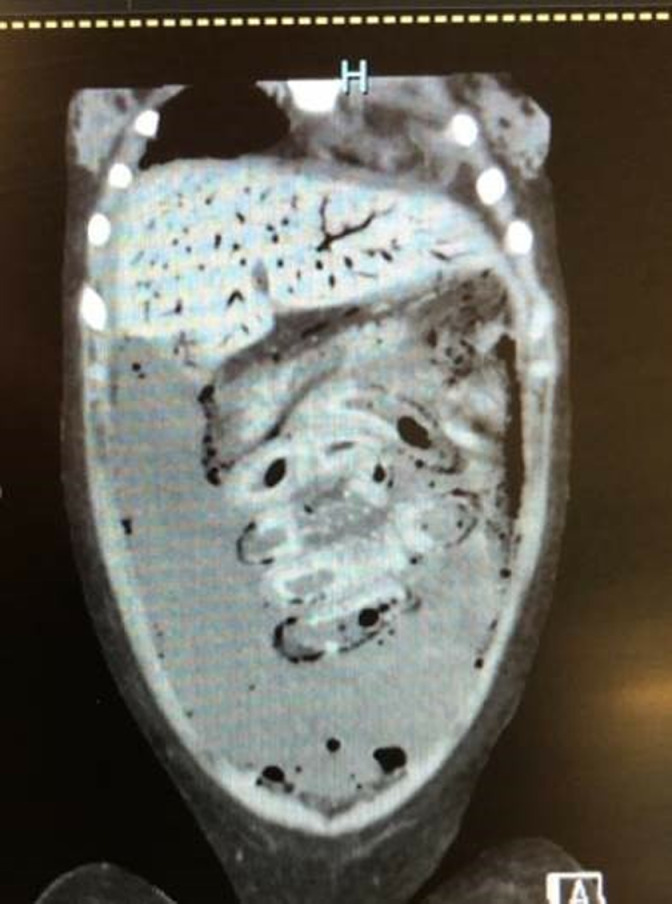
Abdominal CT scan of a 15-year-old girl with abdominal sepsis 1 week after a bone marrow transplant. Hemorrhagic necrosis of the small bowel is seen in addition to extensive pneumatosis intestinalis, ascites and the periportal pneumatosis in the liver. *Clostridium perfringens* was recovered from the blood and ascitic fluid.
